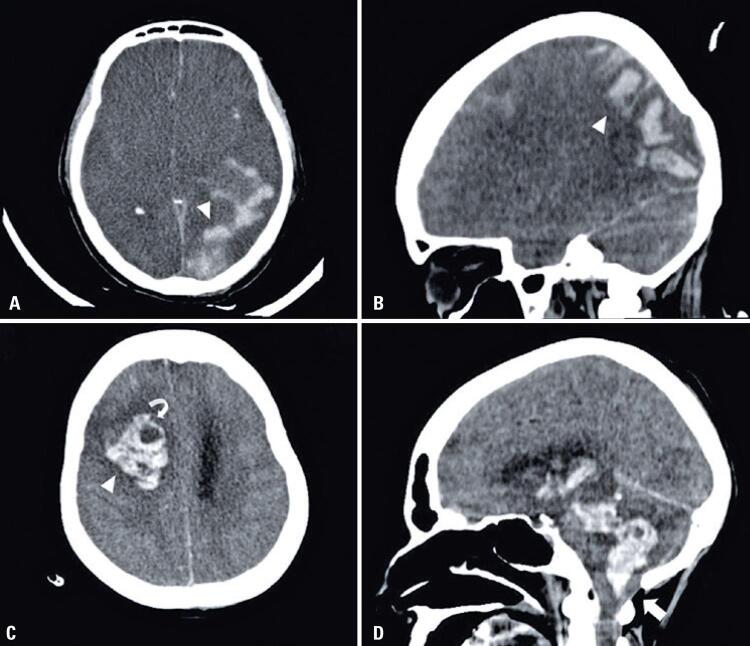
Computed tomography of patients 5 (A) and 6 (B). Diffuse brain edema, loss of gray and white matter differentiation, and large parenchymal hematomas (white arrowhead). Subarachnoid hemorrhage is also present. Computed tomography of patient 7 (C): hemorrhage with heterogeneous attenuation and hypodense areas in between (curved arrow), indicating active bleeding. Reformatted sagittal plane of the patient’s computed tomography scan (D): diffuse edema of the brain parenchyma, with brainstem and cerebellar tonsil herniation, through the foramen magnum (white arrow)
